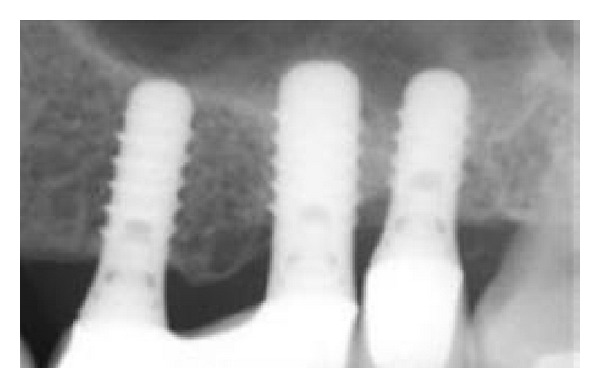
Periapical radiograph after two years of prosthetic loading showing a stable clinical situation in the area around the apex of the implants. A dome-shaped structure was apparent at the sites of the first and second molars.
